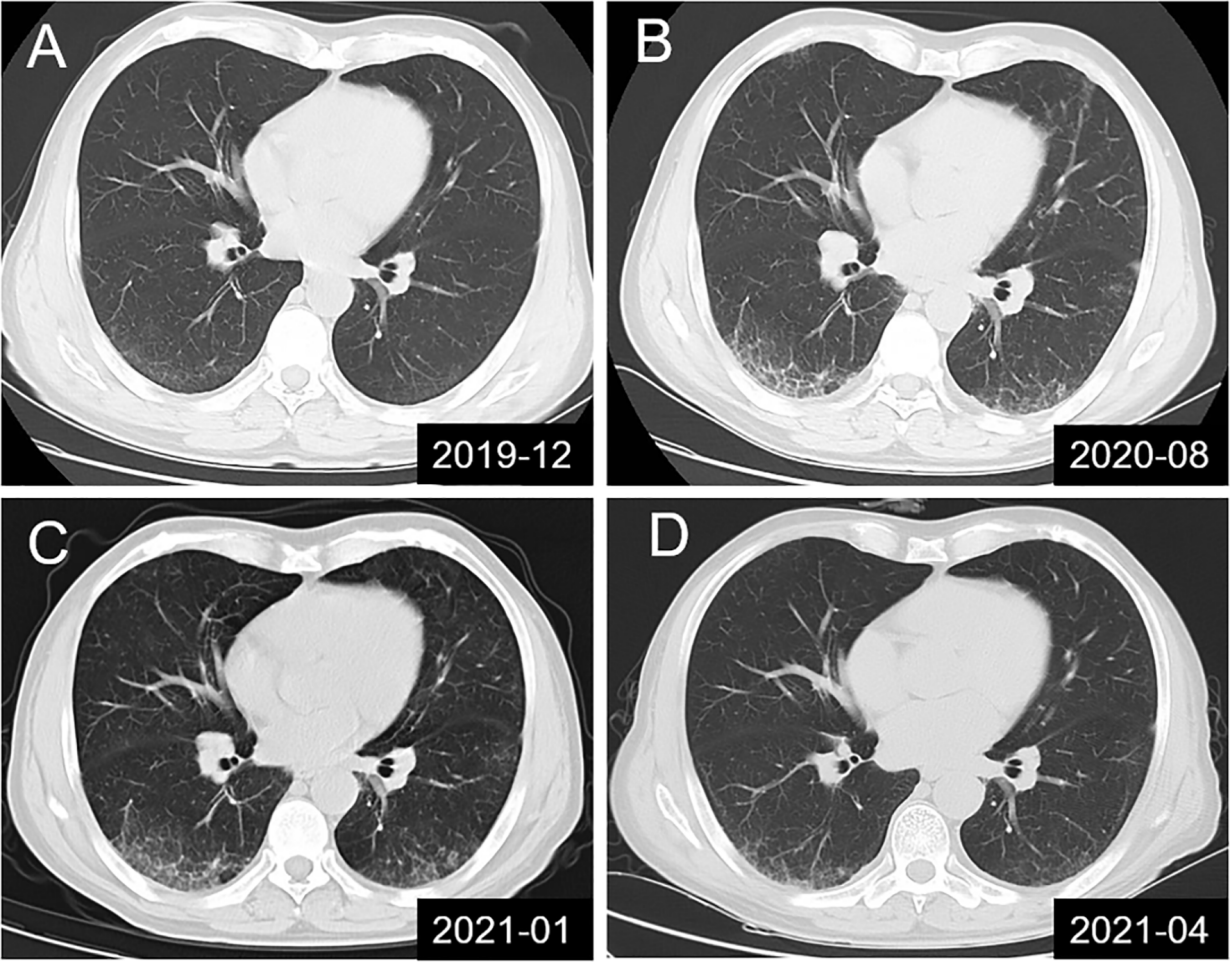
Lung CT scans showing dynamic changes in interstitial pneumonia in the studied patient. (A) Mild interstitial pneumonia found during physical examination; (B, C) Dynamic monitoring before treatment and in the absence of clinical symptoms; (D) Significant improvement in interstitial pneumonia was observed in the results of lung CT re-examination at 10 days after combination therapy with intravenous immunoglobulin, methylprednisolone and rituximab.
